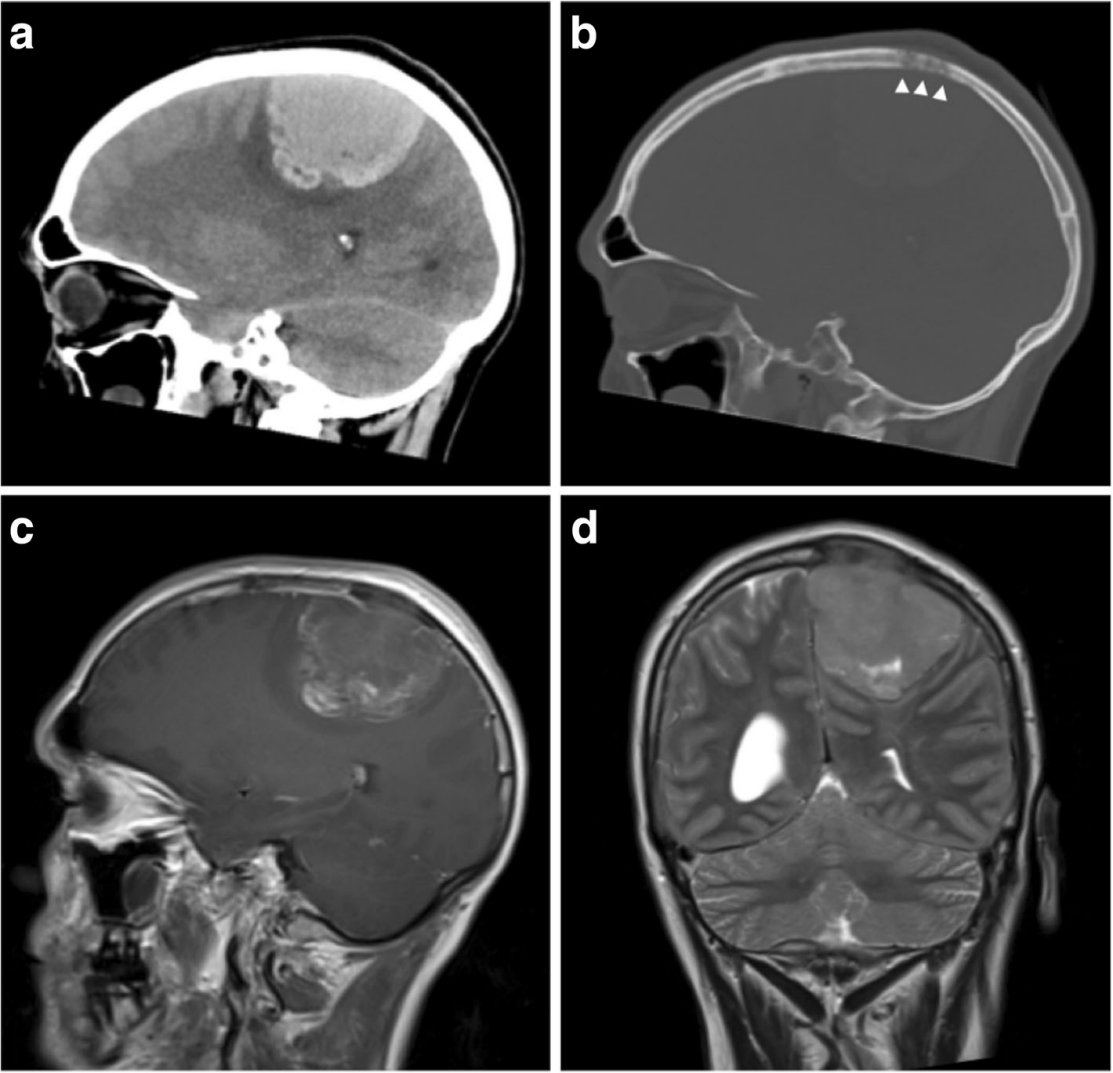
Non-germ cell seminoma metastasis. a Sagittal unenhanced CT image showing an irregular and hyperdense extra-axial mass with a wide dural attachment and only minimal vasogenic oedema. b Sagittal CT image on the bone windowing showing bony destruction adjacent to the lesion (white arrowheads) and no hyperostosis. c Post-contrast sagittal T1-weighted MR image showing only minimal irregular peripheral enhancement and no dural tail. The sagittal sinus is involved and occluded. On unenhanced T1-weighted images, the lesion was isointense to white matter and contained small areas of T1-shortening consistent with haemorrhage. d Coronal T2-weighted image of the same lesion
